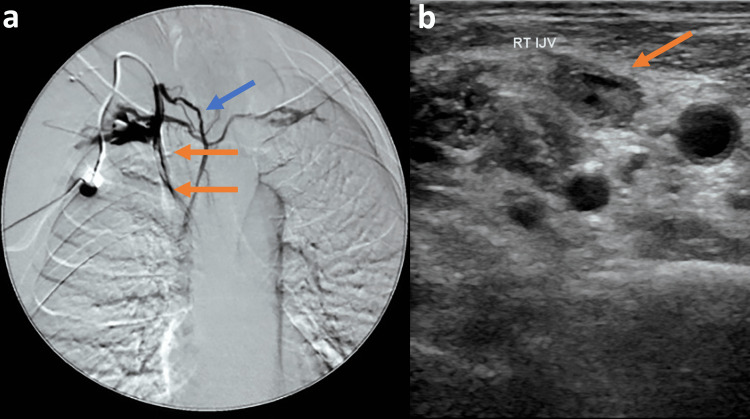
Catheter-related thrombosis a. Venography through the port, revealing right innominate vein and superior vena cava thrombosis (orange arrows), with collateral circulation (blue arrow). b. Ultrasound examination revealing right internal jugular's vein thrombosis with echogenic material in its lumen (orange arrow). RT: right, ijv: internal jugular vein.
